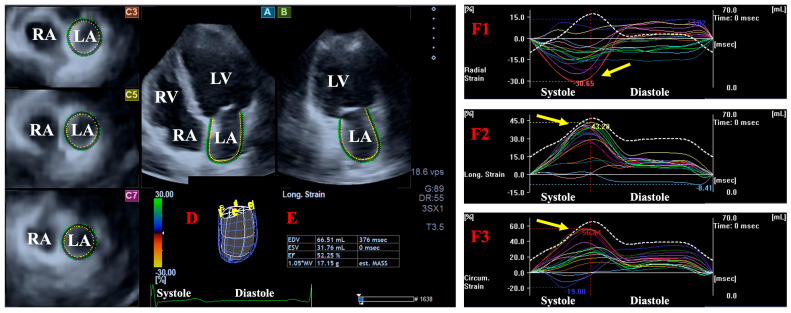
Three-dimensional (3D) speckle-tracking echocardiography-derived determination of left atrial (LA) strains: apical four-chamber (A) and two-chamber (B) long-axis views and short-axis views at basal (C3), mid-atrial (C5), and superior LA levels (C7) are demonstrated together with a virtual 3D model of LA (D) and calculated LA volumetric data (E). Global (white line) and segmental (colored lines) time–radial (F1), –longitudinal (F2), and –circumferential (F3) LA strain curves are presented together with time–LA volume change curves (dashed while line). Yellow arrow represents end-systolic peak LA strains. Abbreviations. EDV, end-diastolic volume; EF, ejection fraction; ESV, end-systolic volume; RA, right atrium; RV, right ventricle; LA, left atrium; LV, left ventricle.
